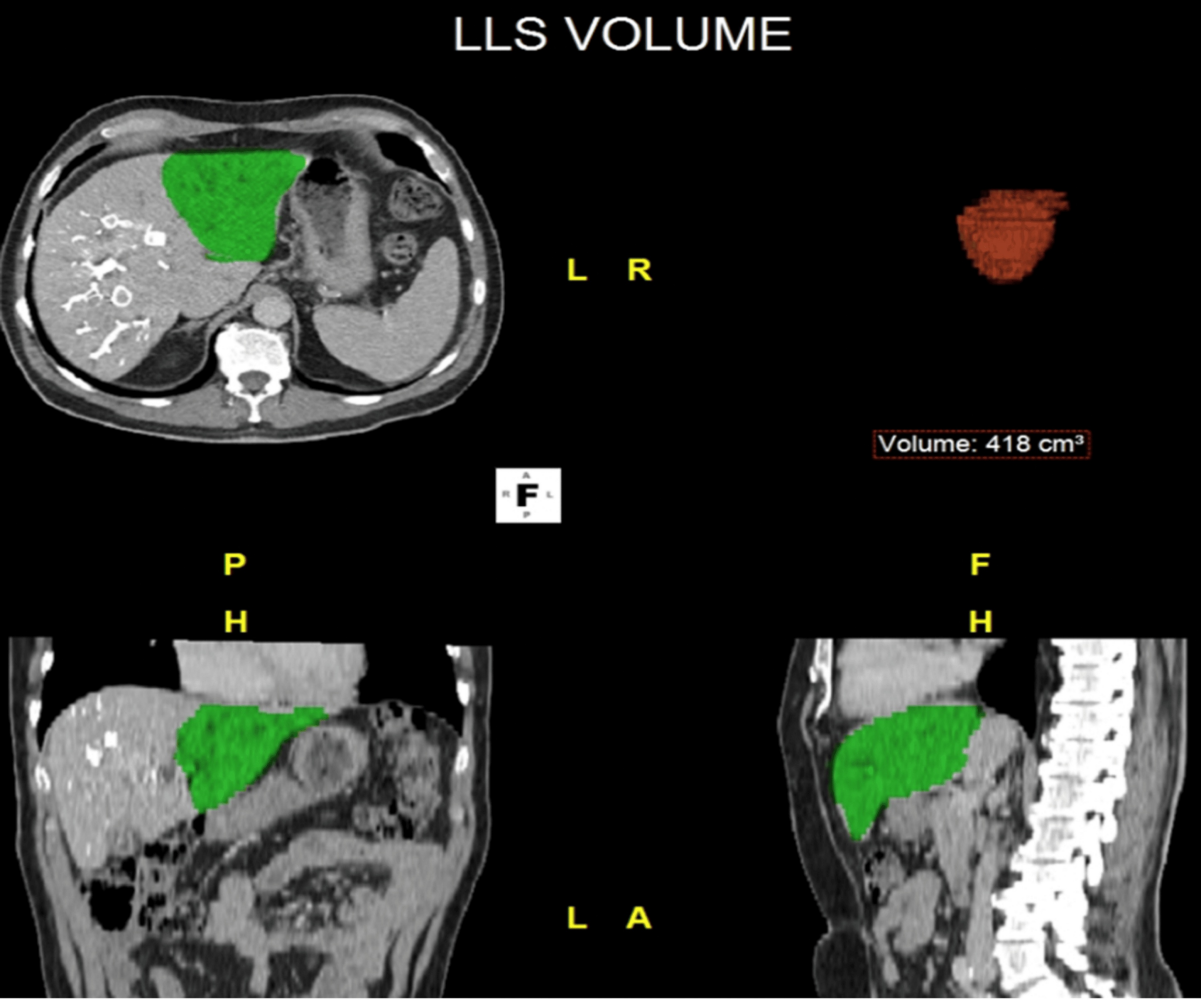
CT-derived volumetry of FLR post LVD Based on CT imaging, volumetric analysis calculates FLR after volume manipulation prior to extended liver resection. In this case, LVD was performed in which the right portal vein as well as the right hepatic veins are occluded to provoke contralateral liver regeneration. The final volume calculation of FLR is 418 cm³. CT: computed tomography, FLR: future liver remnant, LVD: liver vein deprivation
